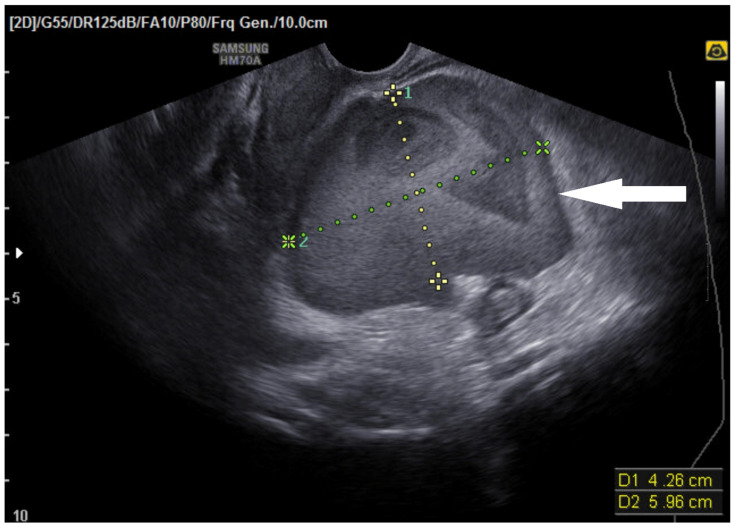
Transabdominal ultrasonography showing a 4.26 × 5.96 cm heterogeneous hematoma adjacent to the left uterine fundus. The arrow indicates the localized hematoma, and the dotted lines represent the measured dimensions.
